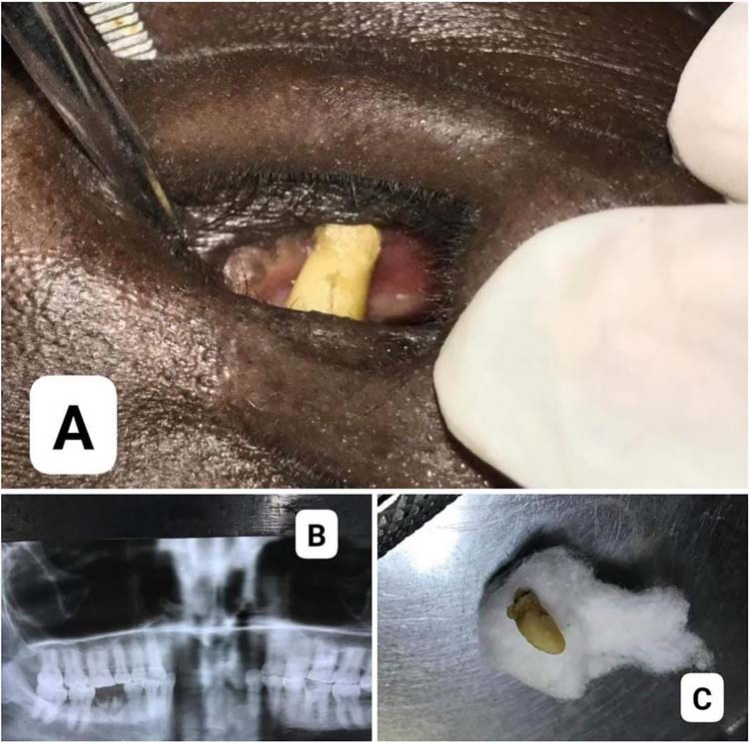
(A) Tooth-like structure protruding from the nasal septum; (B) radiographic appearance of a tooth-like structure in the right nasal cavity; (C) the supernumerary tooth after surgical removal.
